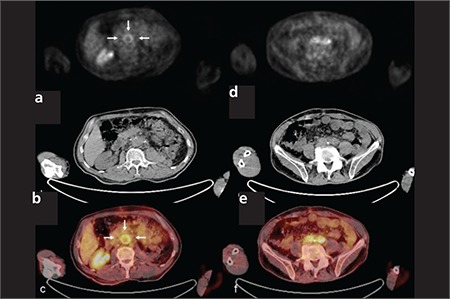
Diffuse increased uptake of FDG on abdominal aorta, rightand left iliac communis arteries on axial PET (a), CT (b) and PET/CTfusion (c) images (SUVmax: 5.4)
